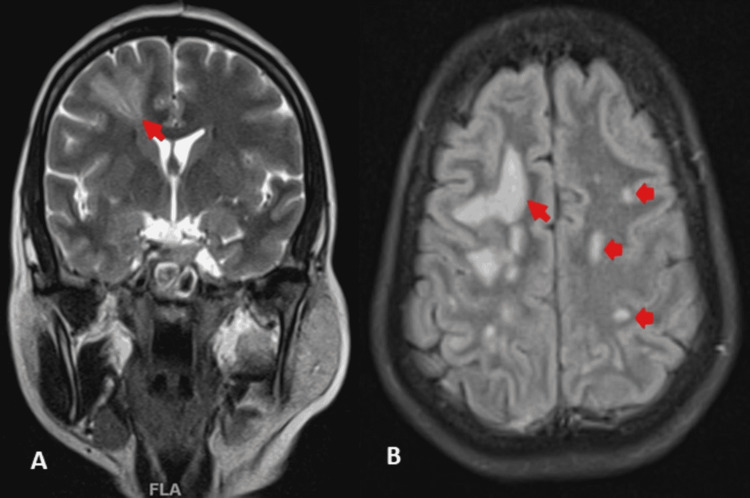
A: Coronal T2 FLAIR MRI of the brain showing T2 hyperintensity predominantly in the right frontal lobe (red arrow), suggestive of a brain abscess. B: Axial T2 FLAIR MRI of the brain showing the same lesion with multiple similar lesions in both cerebral hemispheres.
